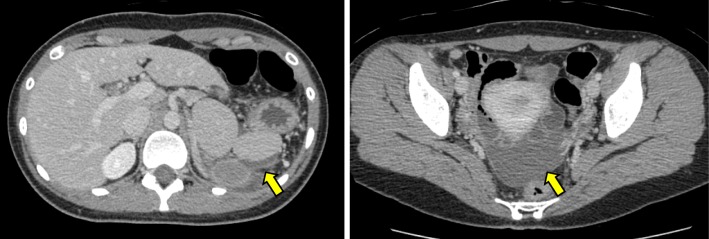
Computed tomography findings 2 days after admission. Ascites is apparent (yellow arrows)
